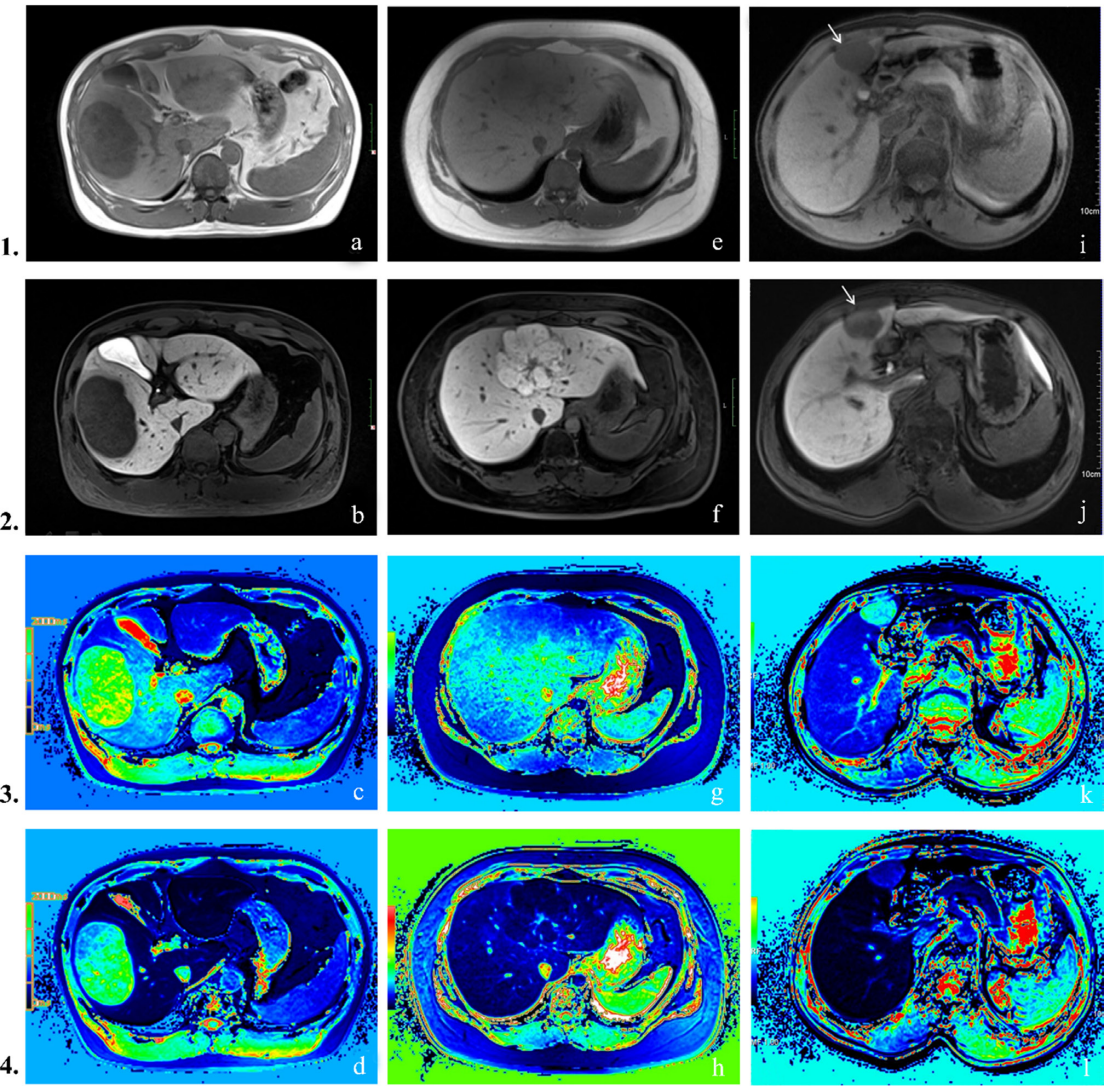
Row 1: pre-contrast T1-weighted images, Row 2: hepatobiliary phase images, Row 3: pre-contrast T1 mapping images, Row 4: hepatobiliary phase T1 mapping images (A–D) Hepatocellular carcinoma. Lesion appeared hypointense on both pre-contrast and hepatobiliary phase images. T1_P_ 712 ms, T1_E_ 529 ms, T1_D_ = 183 ms, T1_D_% = 25.7%. (E–H) Focal nodular hyperplasia. Characteristic findings include central scar and high signal intensity on the hepatobiliary phase. T1_P_ 648 ms, T1_E_ 89 ms, T1_D_ = 559 ms, T1_D_% = 86.3%. (I–L) Cavernous hemangioma of liver. Signal intensities are low in both pre-contrast and hepatobiliary phase T1WI. T1_P_ 1351 ms, T1_E_ 347 ms, T1_D_ = 1004 ms, T1_D_% = 64.3%.
